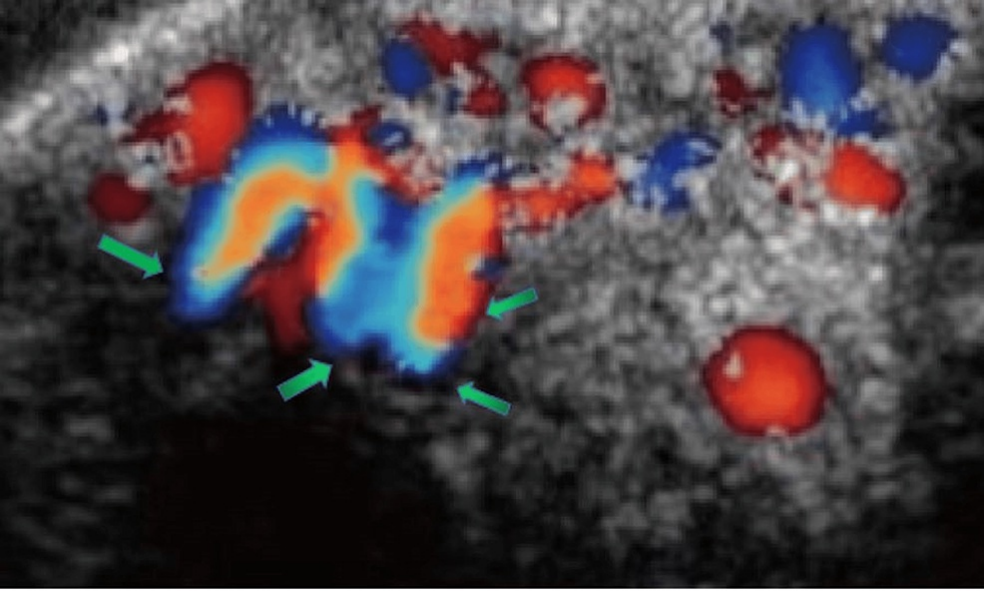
Color Doppler ultrasonography showing a hypoechoic, homogeneous, soft-tissue mass in the midline of the upper lip.
